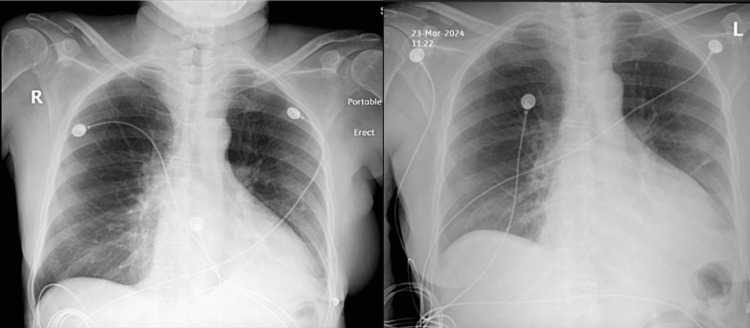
The chest X-ray shows mild bilateral pleural effusion, greater on the left than the right, with moderate collapse/consolidation of the basal segments of the left lower lobe.
